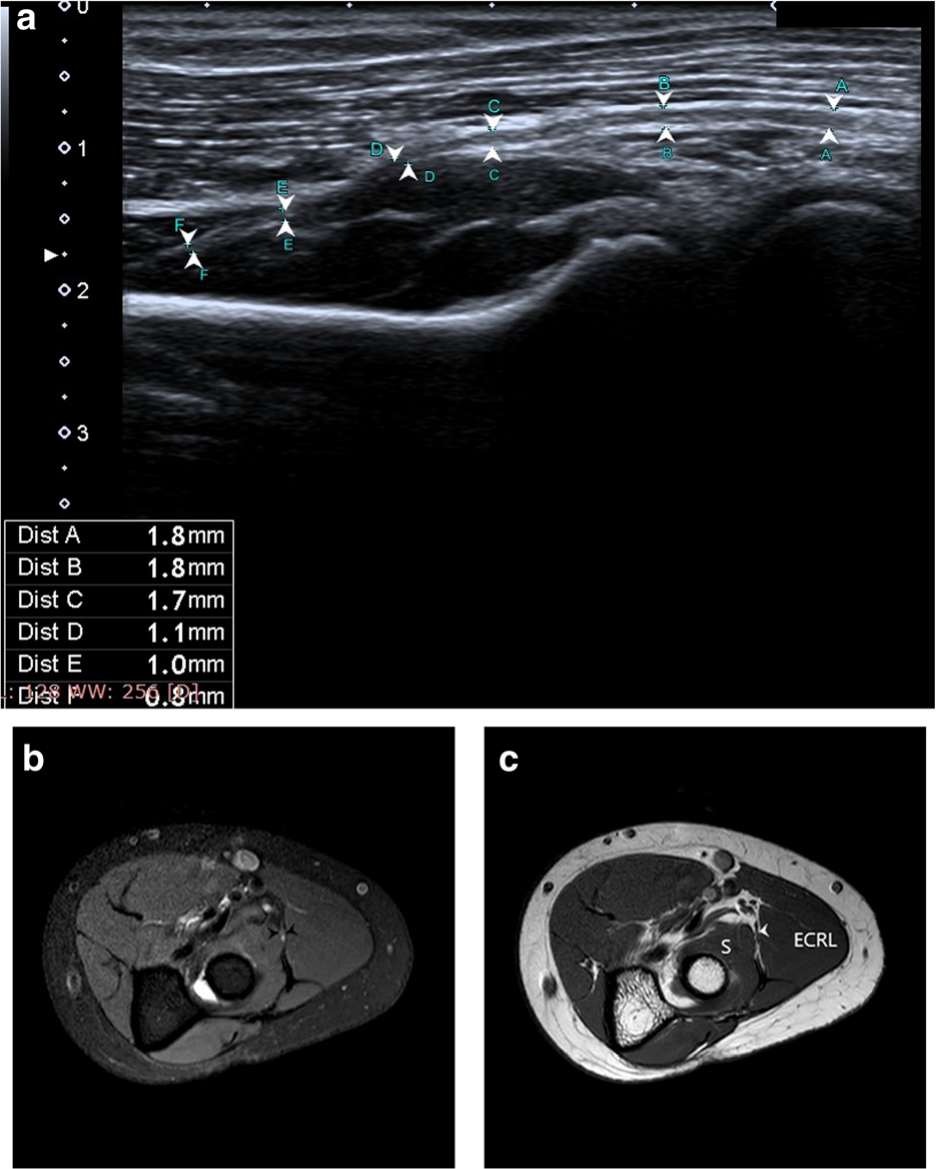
a Longitudinal scan over the radial head shows the PIN (between arrowheads) coursing over the radial head and passing through the radial tunnel. The nerve is hypoechoic and thickened just before passing through the arcade of Frohse in a patient with radial tunnel syndrome. b PD FS axial image shows hyperintense PIN with bulbous swelling just before it pierces the arcade of Frohse, lying between the distal edge of ECRL and superficial supinator. c T1-weighted axial image shows the course of the PIN soon after its division from the radial nerve passing between the ECRL and supinator. The diagnosis of radial tunnel syndrome was confirmed in this patient by giving ultrasound-guided PIN block [22]
